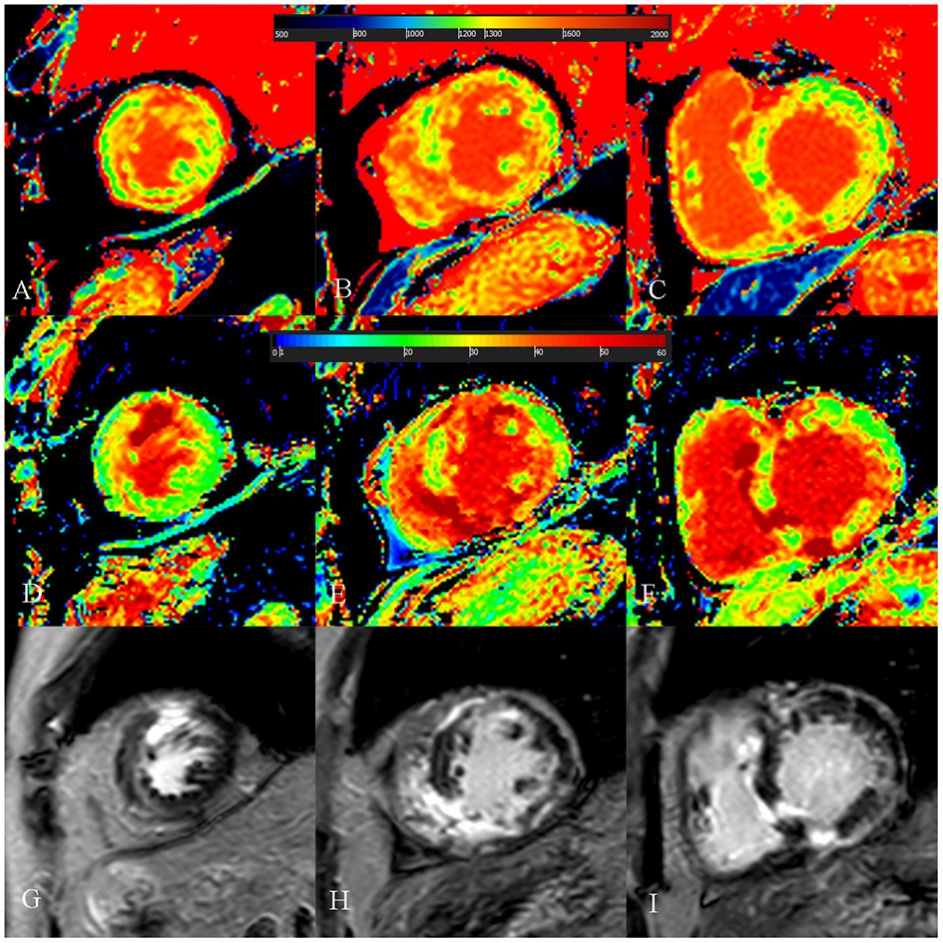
A 52-year-old male with burned out phase hypertrophic cardiomyopathy. (A–C) Native T1 mapping of the apical, mid-, and basal portions of the left ventricular (LV) myocardium revealed higher global native T1 (1,380.7 ms). (D–F) Extracellular volume fraction (ECV) of the apical, mid-, and basal portions of the LV myocardium showed higher global ECV (32.8%). (G–I) Late gadolinium enhancement (LGE) images of the apical, mid-, and basal portions of the LV myocardium showed multiple LGE. The maximal LV wall thickness was 13 mm, left atrial diameter was 53 mm, and the LV outflow tract gradient pressure was 2 mmHg. He had non-sustained ventricular tachycardia, but no family history of sudden cardiac death (SCD), and unexplained syncope. He had LGE/LV mass ≥15% (elevated LGE/LV mass: 56.2%) and end-stage systolic dysfunction but no apical aneurysm. He was stratified with low SCD risk under the 2014 European Society of Cardiology guidelines (5-year SCD probability: 3.39%) and high SCD risk under the enhanced American College of Cardiology/American Heart Association guidelines. He underwent cardiac transplantation 35 months after cardiac MRI examination. End-stage systolic dysfunction strongly indicated poor outcome in this patient. However, increased global native T1 mapping and ECV values could also suggest that the patient was likely to be at high risk of SCD and needs further treatment.
